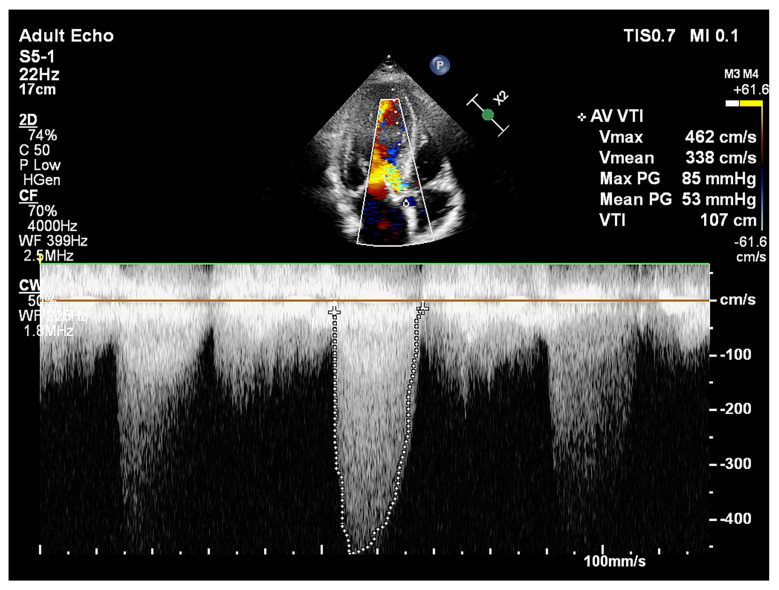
Echocardiographic assessment of a symptomatic patient with AS and SHM. The image demonstrates increased transvalvular aortic gradients and turbulent blood flow in the left ventricular outflow tract. That the turbulent flow directly contacts the predominant septal base, which may contribute to SHM development under chronic AS-mediated stress.
